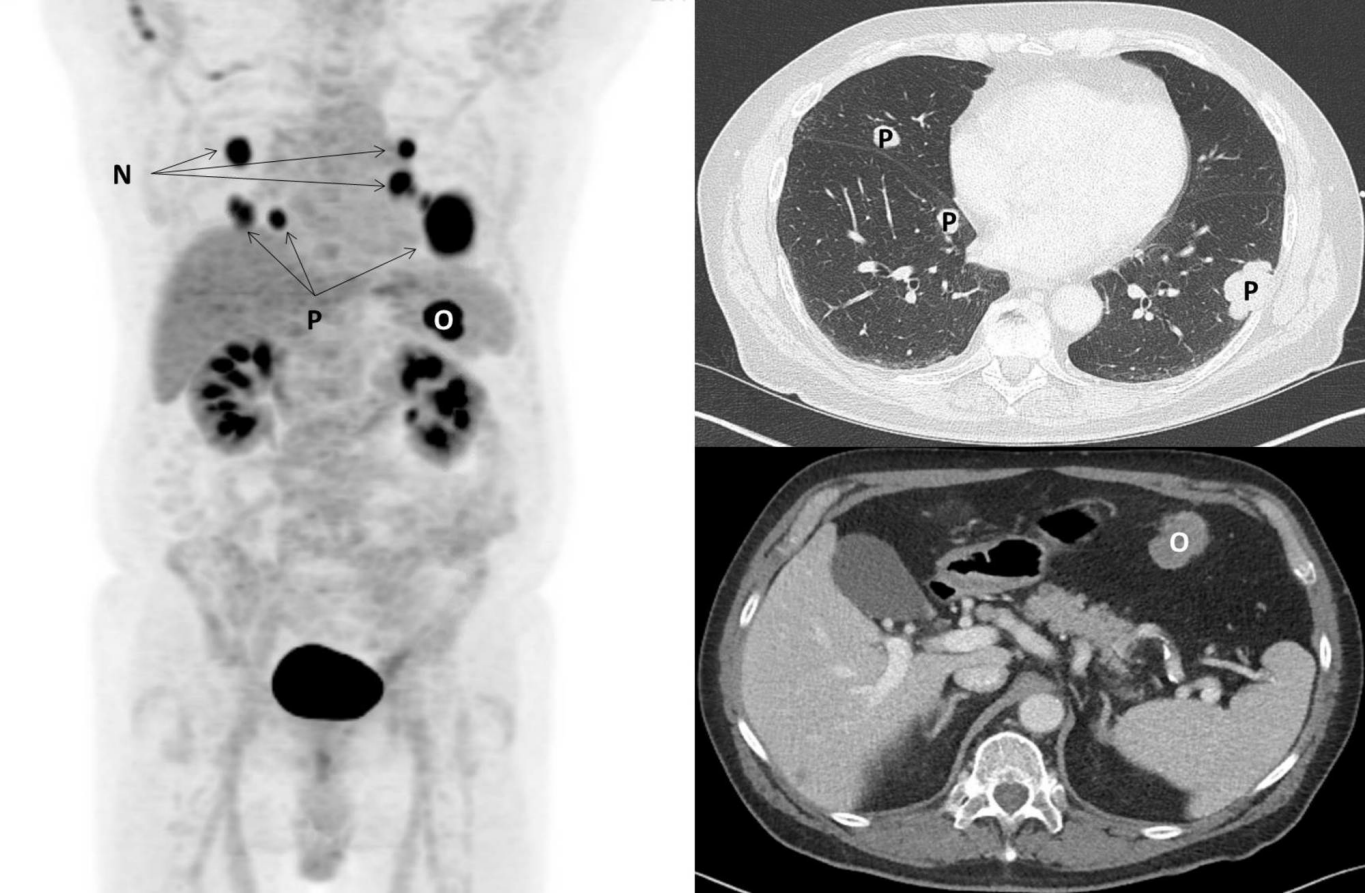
Staging imaging at diagnosis. PET/CT maximum intensity projection (left), and representative transverse CT chest (right, top) and CT abdomen (right, bottom) images are shown. Multiple FDG-avid bilateral hilar lymph nodes (N; left) and pulmonary parenchymal nodules (P; left, and right, top) are seen. An FDG-avid omental mass (O; left, and right, bottom) is seen. PET/CT: Positron emission tomography/computed tomography; FDG: Fluorodeoxyglucose.
